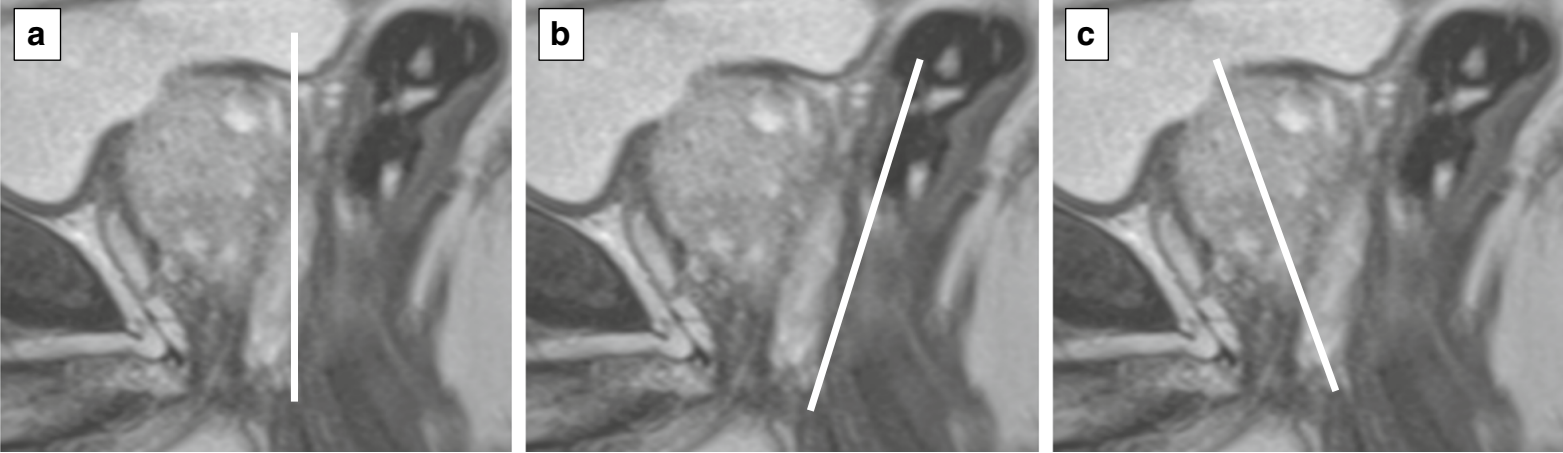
Sagittal T2-weighted acquisitions showing different positions of the axial plane: perpendicular to the MR table/patient (a), orthogonal to the rectum and posterior aspect of the prostate (b) or to the long axis of the prostate (c)
